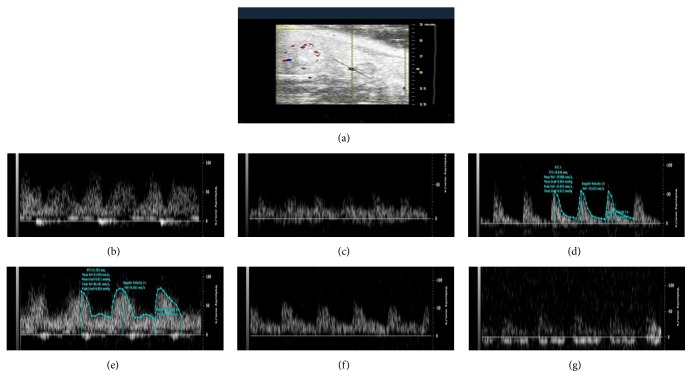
Representative Doppler waveforms of the ovarian artery. (a) The image of the ovary; (b) the control group; (c) the model group; (d) the aspirin group; (e) the DZD-H group; (f) the DZD-M group; (g) the DZD-L group.
